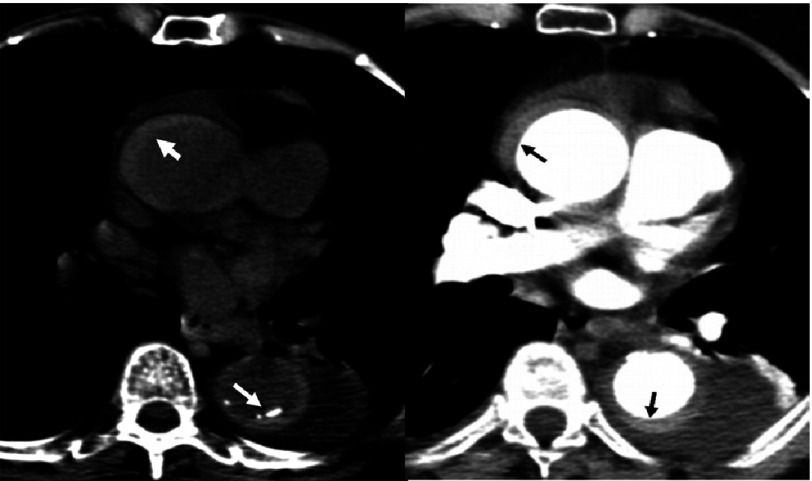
Intramural haematoma. Left) non-enhanced CT showing a high attenuation signal in the aortic wall of the ascending and descending aorta (white arrows); right) CT angiography showing the same intramural haematoma but with more difficulties (black arrows).
